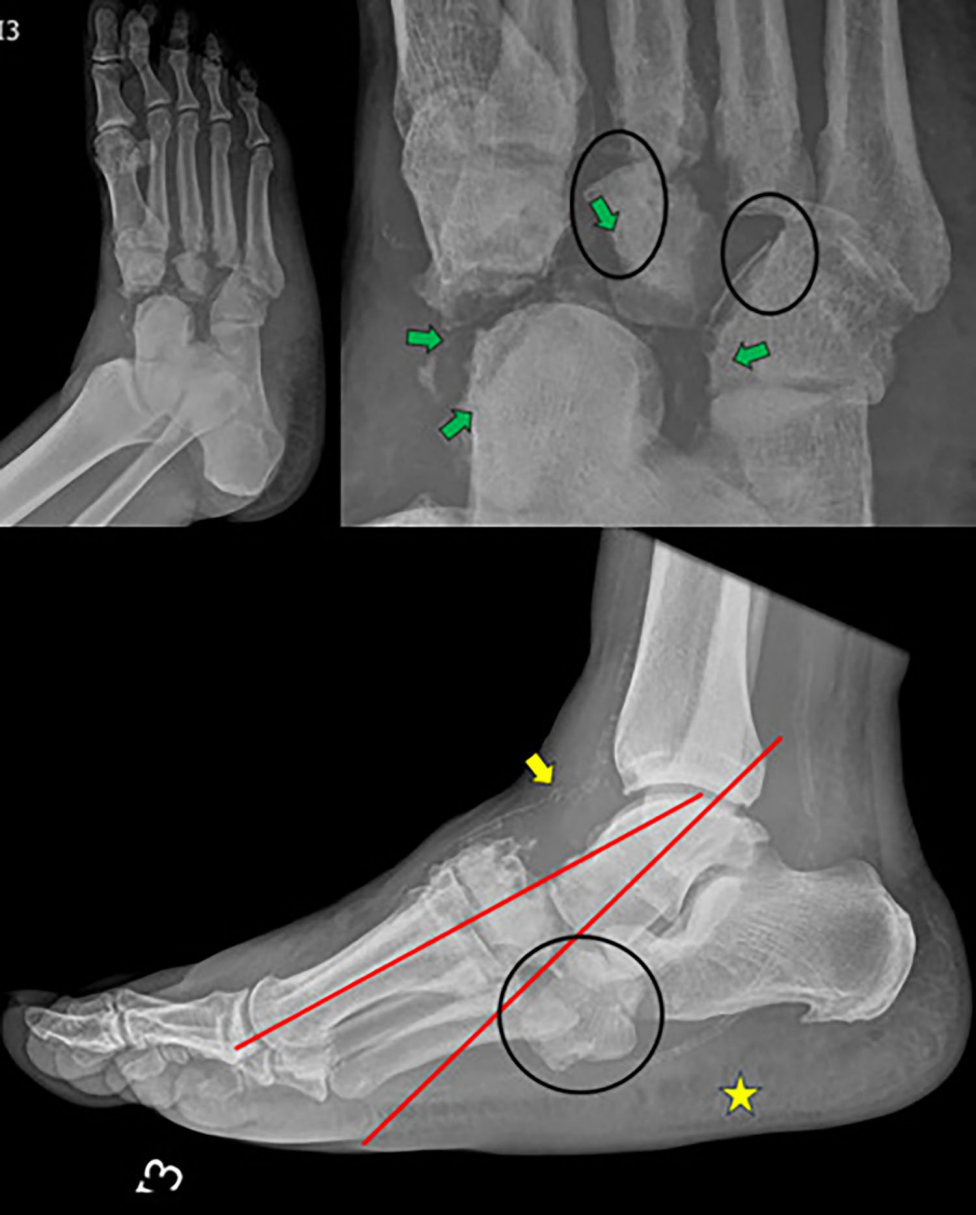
Conventional radiographs of the left foot in oblique projection, magnification of the oblique projection with emphasis on the midfoot, and lateral projection of a 46-year-old man with a history of type 2 diabetes mellitus who presented with 15 days of right foot pain associated with a plantar lesion. The patient had findings compatible with neuropathic atropathy of the foot or Charcot’s atropathy in the fragmentation phase or acute neuropathic arthropathy. The following is the ABCDE systematic approach to plain radiography: A: Subluxation of the talonavicular joint and 3rd wedge cuboid metatarsal (straight red lines). B: Focal osteopenia of the midfoot, subchondral cysts and large osteophytes (surrounded by black circles). C: Intra-articular bony bodies (indicated by green arrows). D: Midfoot involvement. E: Erosions. S: Soft tissue oedema (indicated by the yellow star). Calcification of the pedicle artery (indicated by the yellow arrow) is also seen
